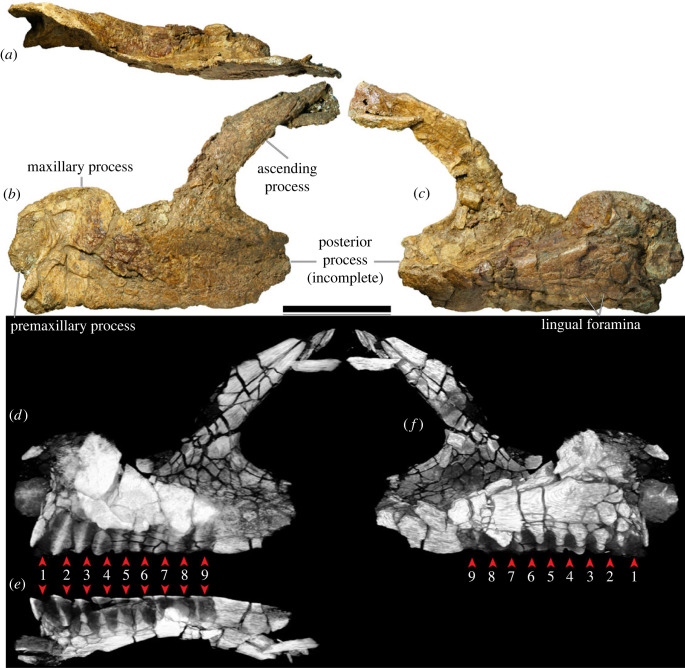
Diamantinasaurus matildae* referred left maxilla (AODF 0906). (*a–c*) Photographs of the left maxilla in (*a*) dorsal, (*b*) lateral and (*c*) medial views. (*d–f*) three-dimensional digital models (derived from medical CT data) of the left maxilla in (*d*) lateral, (*e*) ventral and (*f*) medial views. Numbers 1–9 indicate alveoli. Scale bar = 50 mm.
